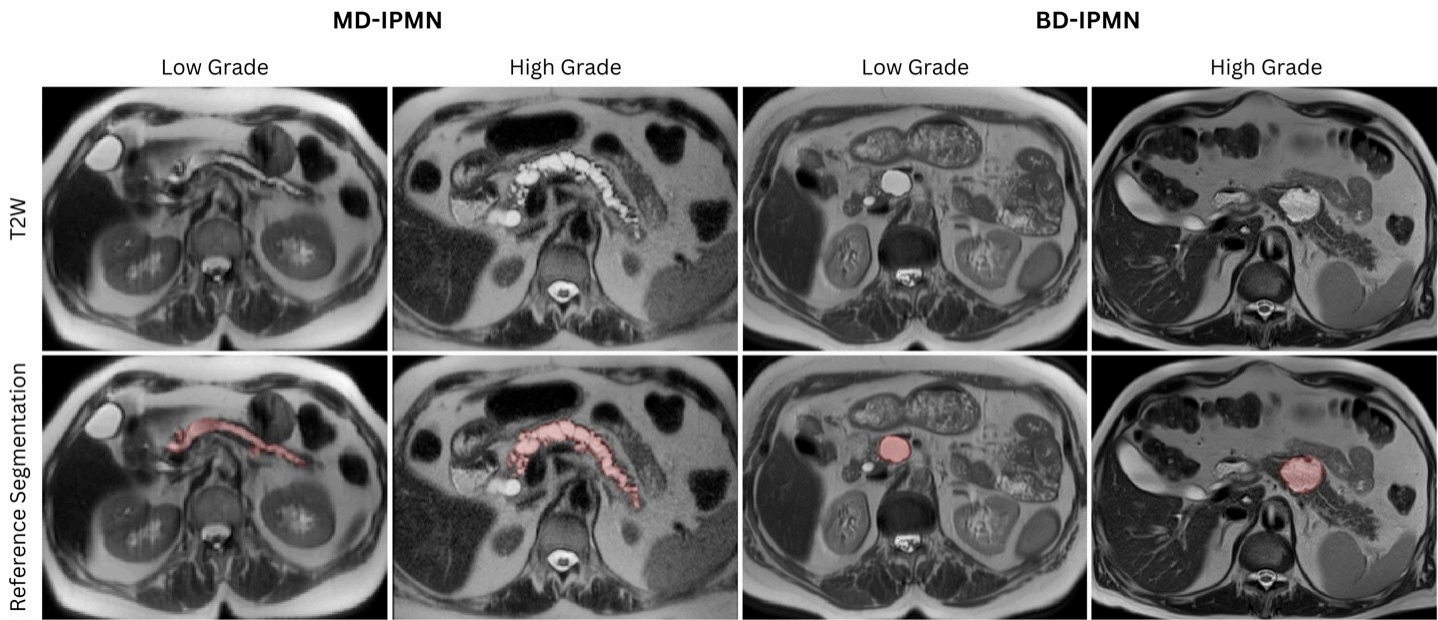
Representative T2-weighted (T2W) images and reference segmentations of high-grade and low-grade IPMNs. The first row shows T2W MRI images, and the second row shows reference segmentations of high-grade and low-grade, main-duct (MD)-IPMN and branch-duct (BD)-IPMN cases.
